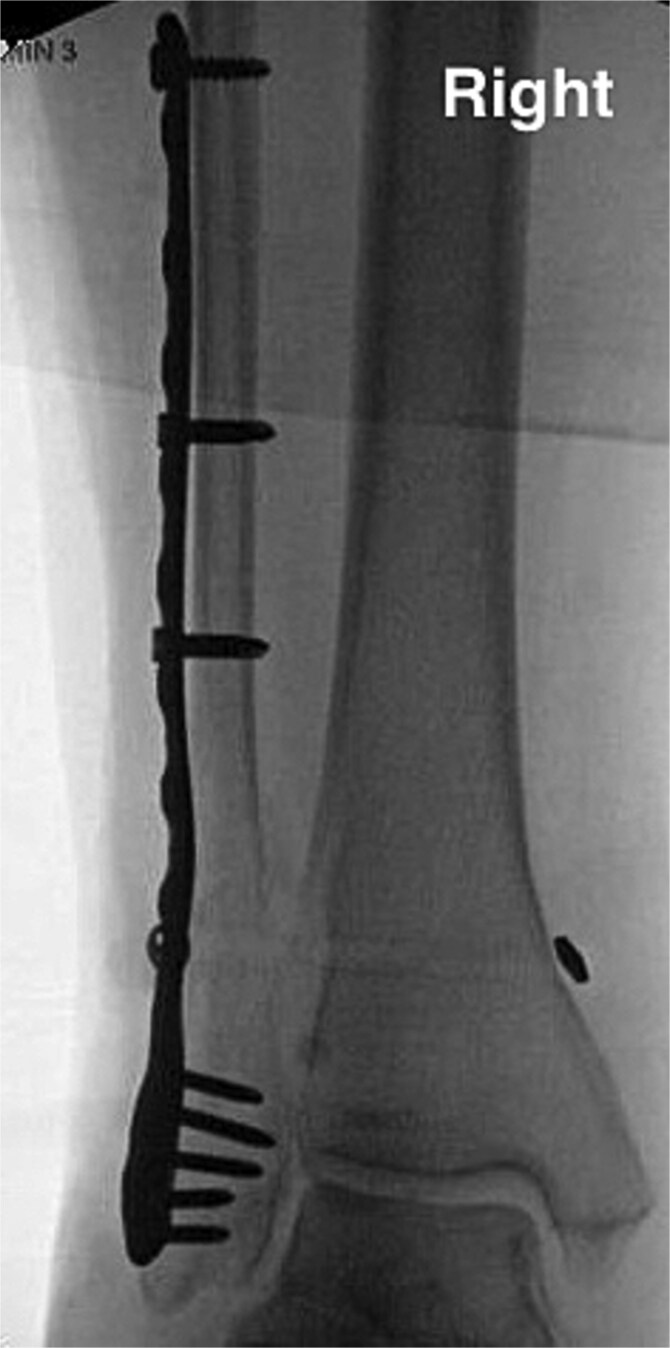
Anterior–posterior post-operative X-ray demonstrating fixation of right Weber C fibular fracture.
